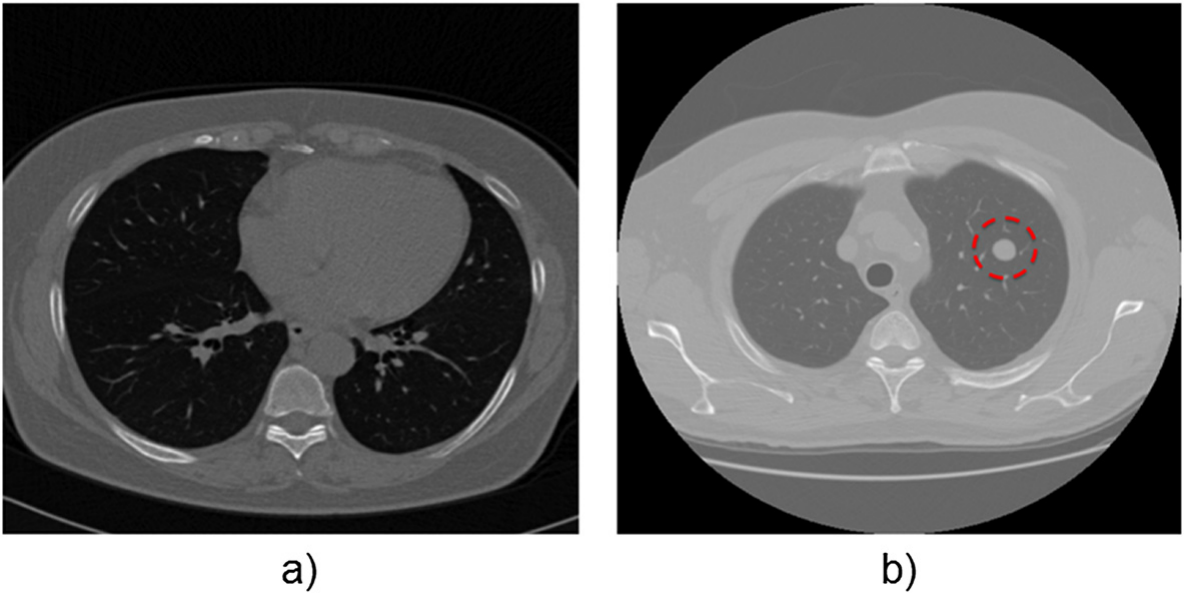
Original CT thorax scan images: a) Without lung nodules and b) With lung nodules. Both images were obtained from LIDC database. On the right the CT image presents a lung nodule with a diameter of 3 mm. The nodule is highlighted with a red dashed circle.
